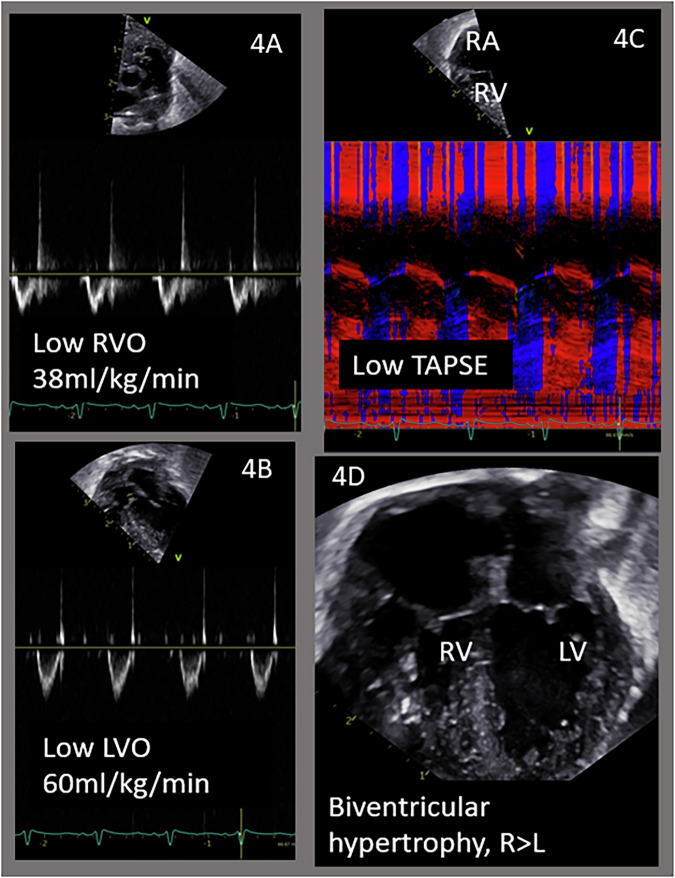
TTTS recipient twin case, selected echocardiography clips. A RVO- right ventricular output. B LVO- left ventricular output. C TAPSE- tricuspid annular plan systolic excursion. D ventricular hypertrophy. RV right ventricle, LV left ventricle.
